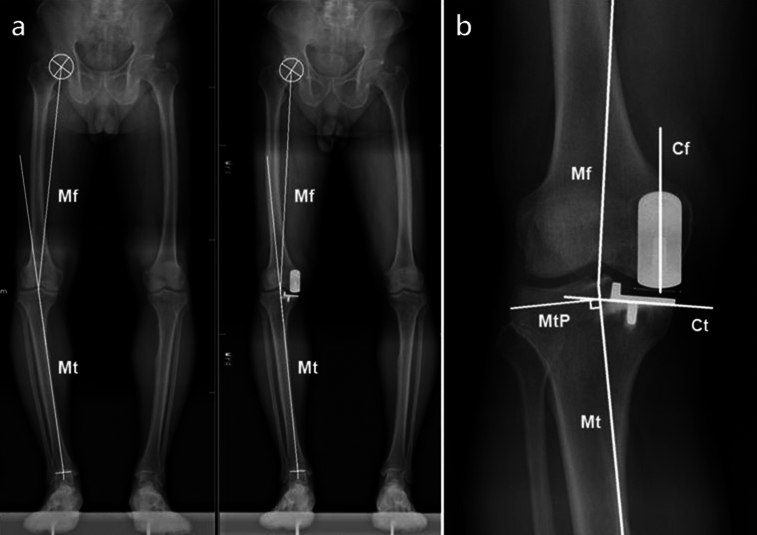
Measurement of preoperative and postoperative hip–knee–ankle angle (a) and component alignment angle (b) on full-length standing anteroposterior radiographs. The hip–knee–ankle angle was defined as the angle between the Line Mf and the Line Mt. The femoral component alignment angle was defined as the angle between the Line Mf and the Line Cf. The tibial component alignment angle was defined as the angle between the Line MtP and the Line Ct. The varus and valgus alignments of the HKA angle, femoral component, and tibial component were defined as positive and negative, respectively.Line Mf, the mechanical axis of the femur; Line Mt, the mechanical axis of the tibia; Line MtP, the perpendicular line of the mechanical axis of the tibia; Line Cf, the longitudinal axis of the femoral component; Line Ct, the undersurface of the tibial component.
